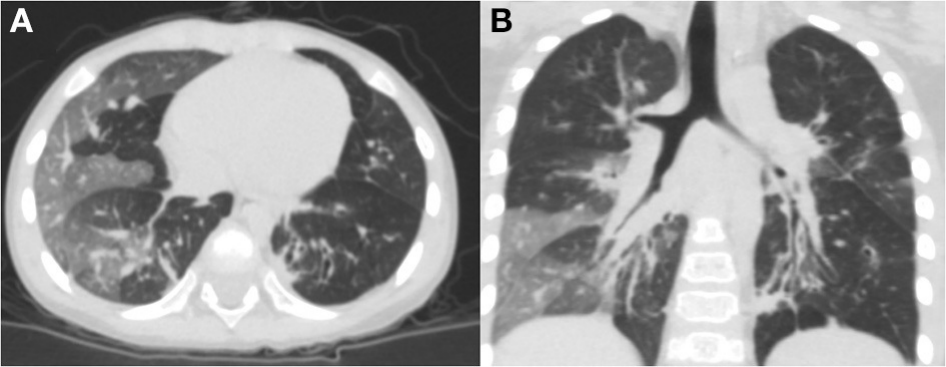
Chest HRCT scans of a 2-year-old boy with PIBO. (A) Mosaic perfusion pattern. (B) Bronchial wall thickening and bronchial dilation (3D reconstruction).
